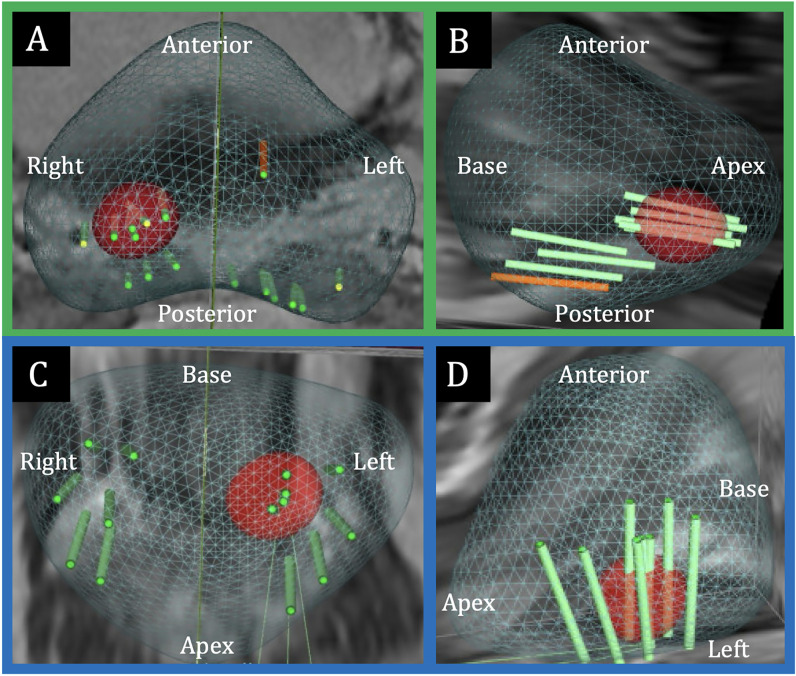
Prostate biopsy templates. A) Axial and B) right sagittal view of the transperineal MRI/TRUS fusion-guided prostate biopsy template. C) Coronal and D) left sagittal view of the transrectal MRI/TRUS fusion-guided prostate biopsy template.
